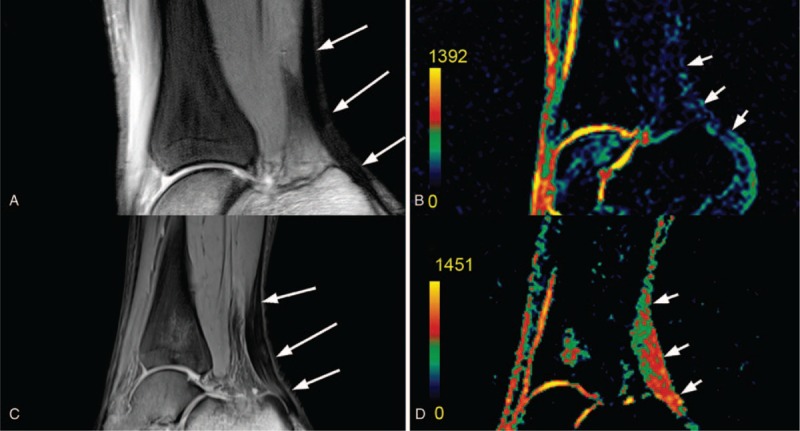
MR images in a 27-year-old healthy volunteer. A, Proton-density-weighted, 2-dimensional, turbo spin-echo image depicts the centers of each ROI (arrows). B, Corresponding color-coded, 3-dimensional, gradient-echo sodium image depicts Achilles tendon sodium signal (arrows), which is minimal. MR images in a 46-year-old patient with chronic Achilles tendinopathy. C, Proton-density-weighted, 2-dimensional, turbo spin-echo image depicts centers of each ROI (arrows). D, Corresponding color-coded, 3-dimensional, gradient-echo sodium image depicts Achilles tendon sodium signal (arrows), which is obviously higher than that in the healthy volunteer. The sodium signal increase was observed in the whole tendon, and not only in regions with clinical findings (middle region in this case). All patients had focal thickening of the Achilles tendon without a fluid-like signal intensity increase on proton-density-weighted, 2-dimensional, turbo spin-echo images. The color scale represents sodium signal intensity values. Reprinted with permission from *Radiology* 2012; 262:199–205.
